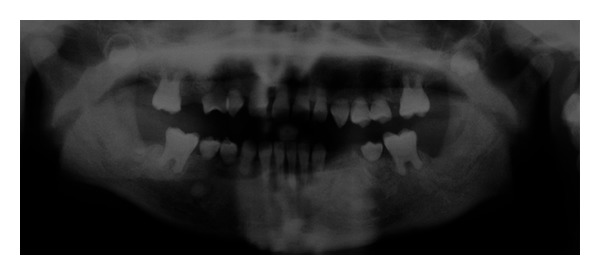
Panoramic radiographic view of the patient at last visit.
